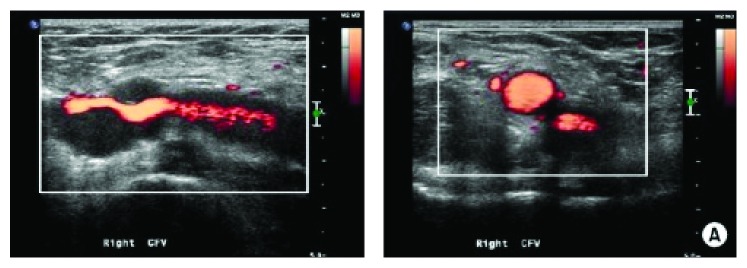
Ultrasound imaging shows a typical hypoechoic fluid-filled cyst with a posterior acoustic window [3].
